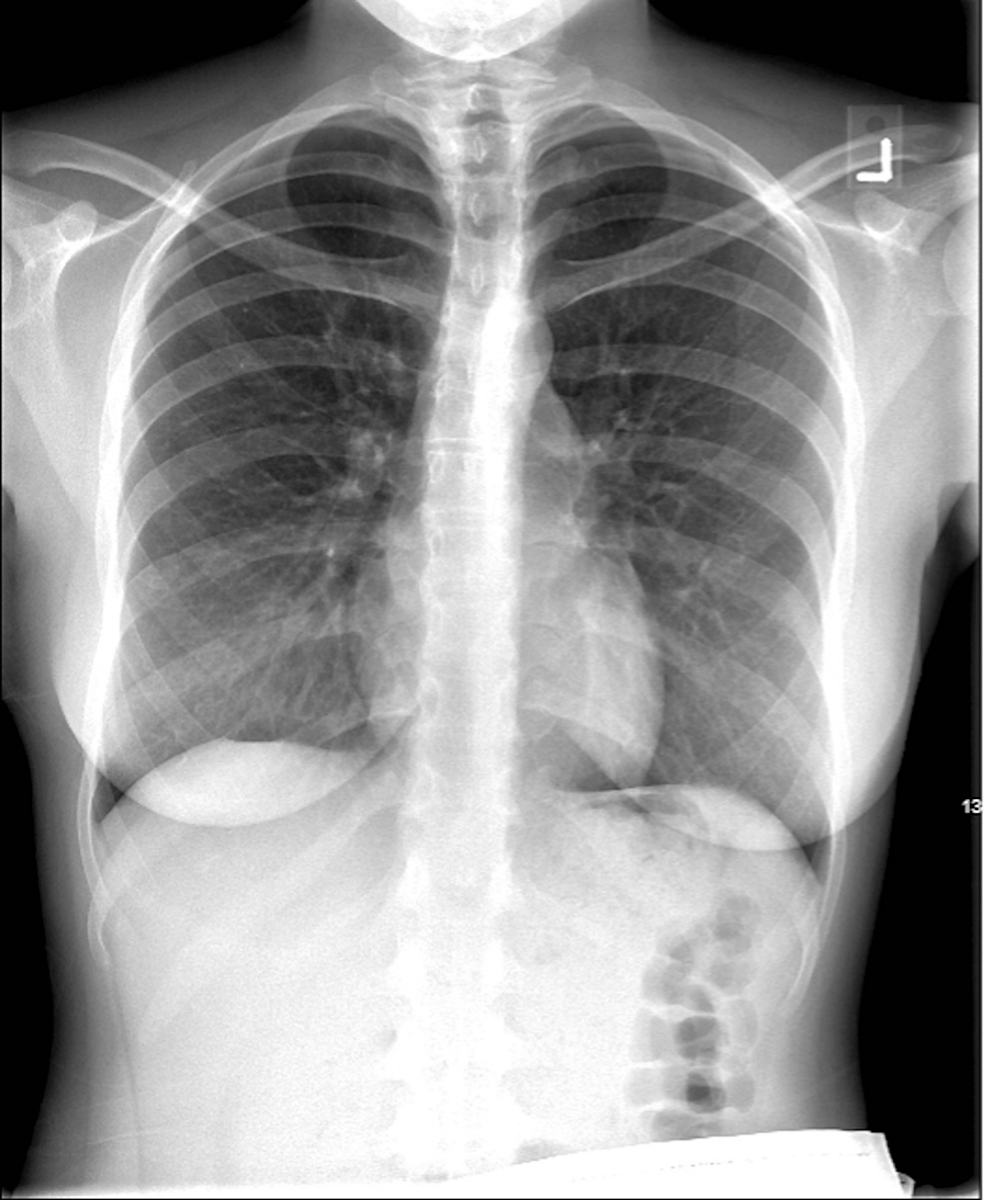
Posteroanterior (PA) chest x-ray reveals no abnormalities
